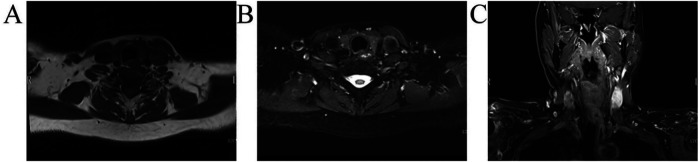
Postoperative imaging of the neck lesion at 28 months. T1-weighted imaging of axial section (A); T2-weighted imaging with DIXON fat suppression of axial section (B); enhanced T1-weighted imaging with DIXON fat suppression of coronal section (C).
